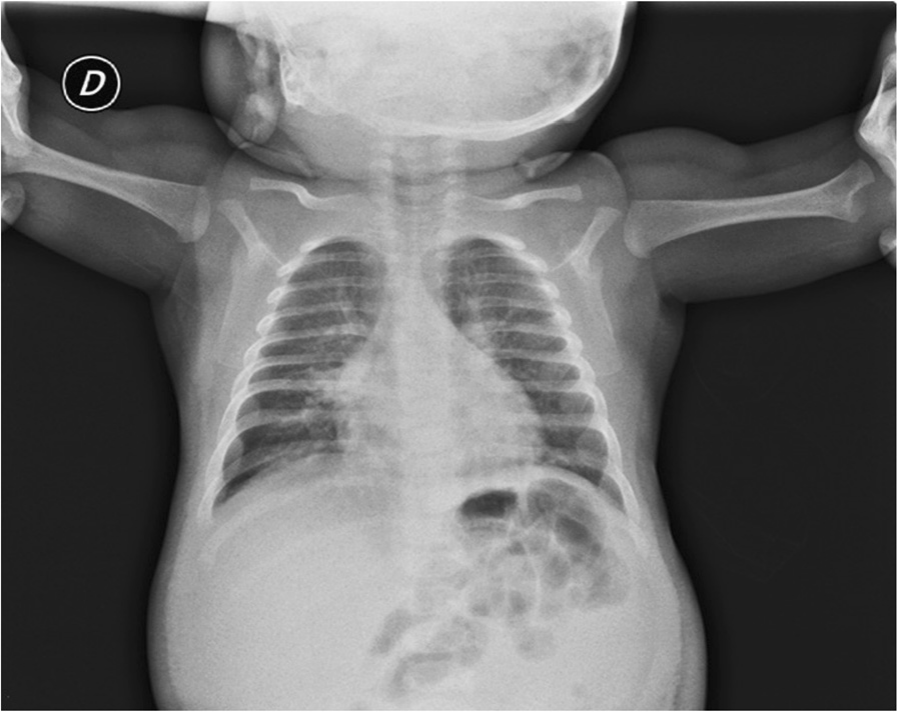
Chest radiography. Generalized increased translucency of the thorax. The heart size was within normal limits.
